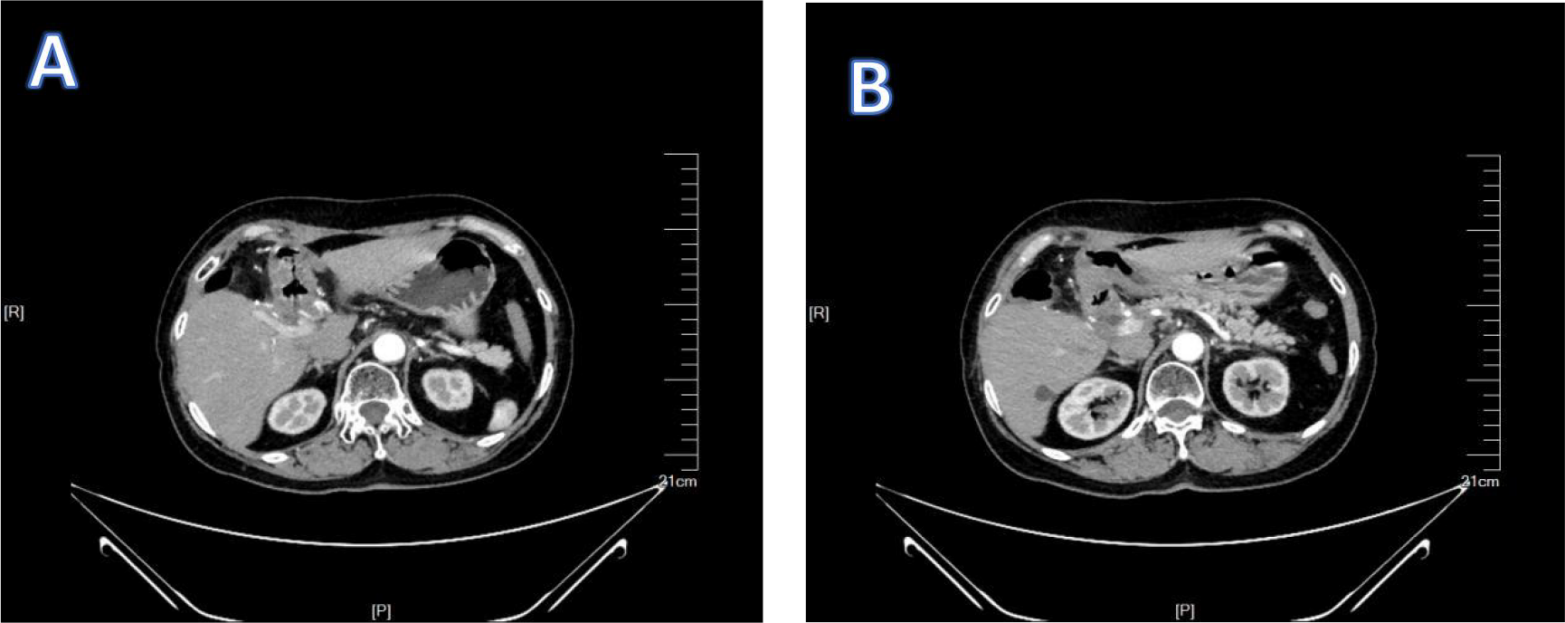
1Postoperative examination of patient 2. (A, B) show the CT examination results of patient 2 after the operation.
